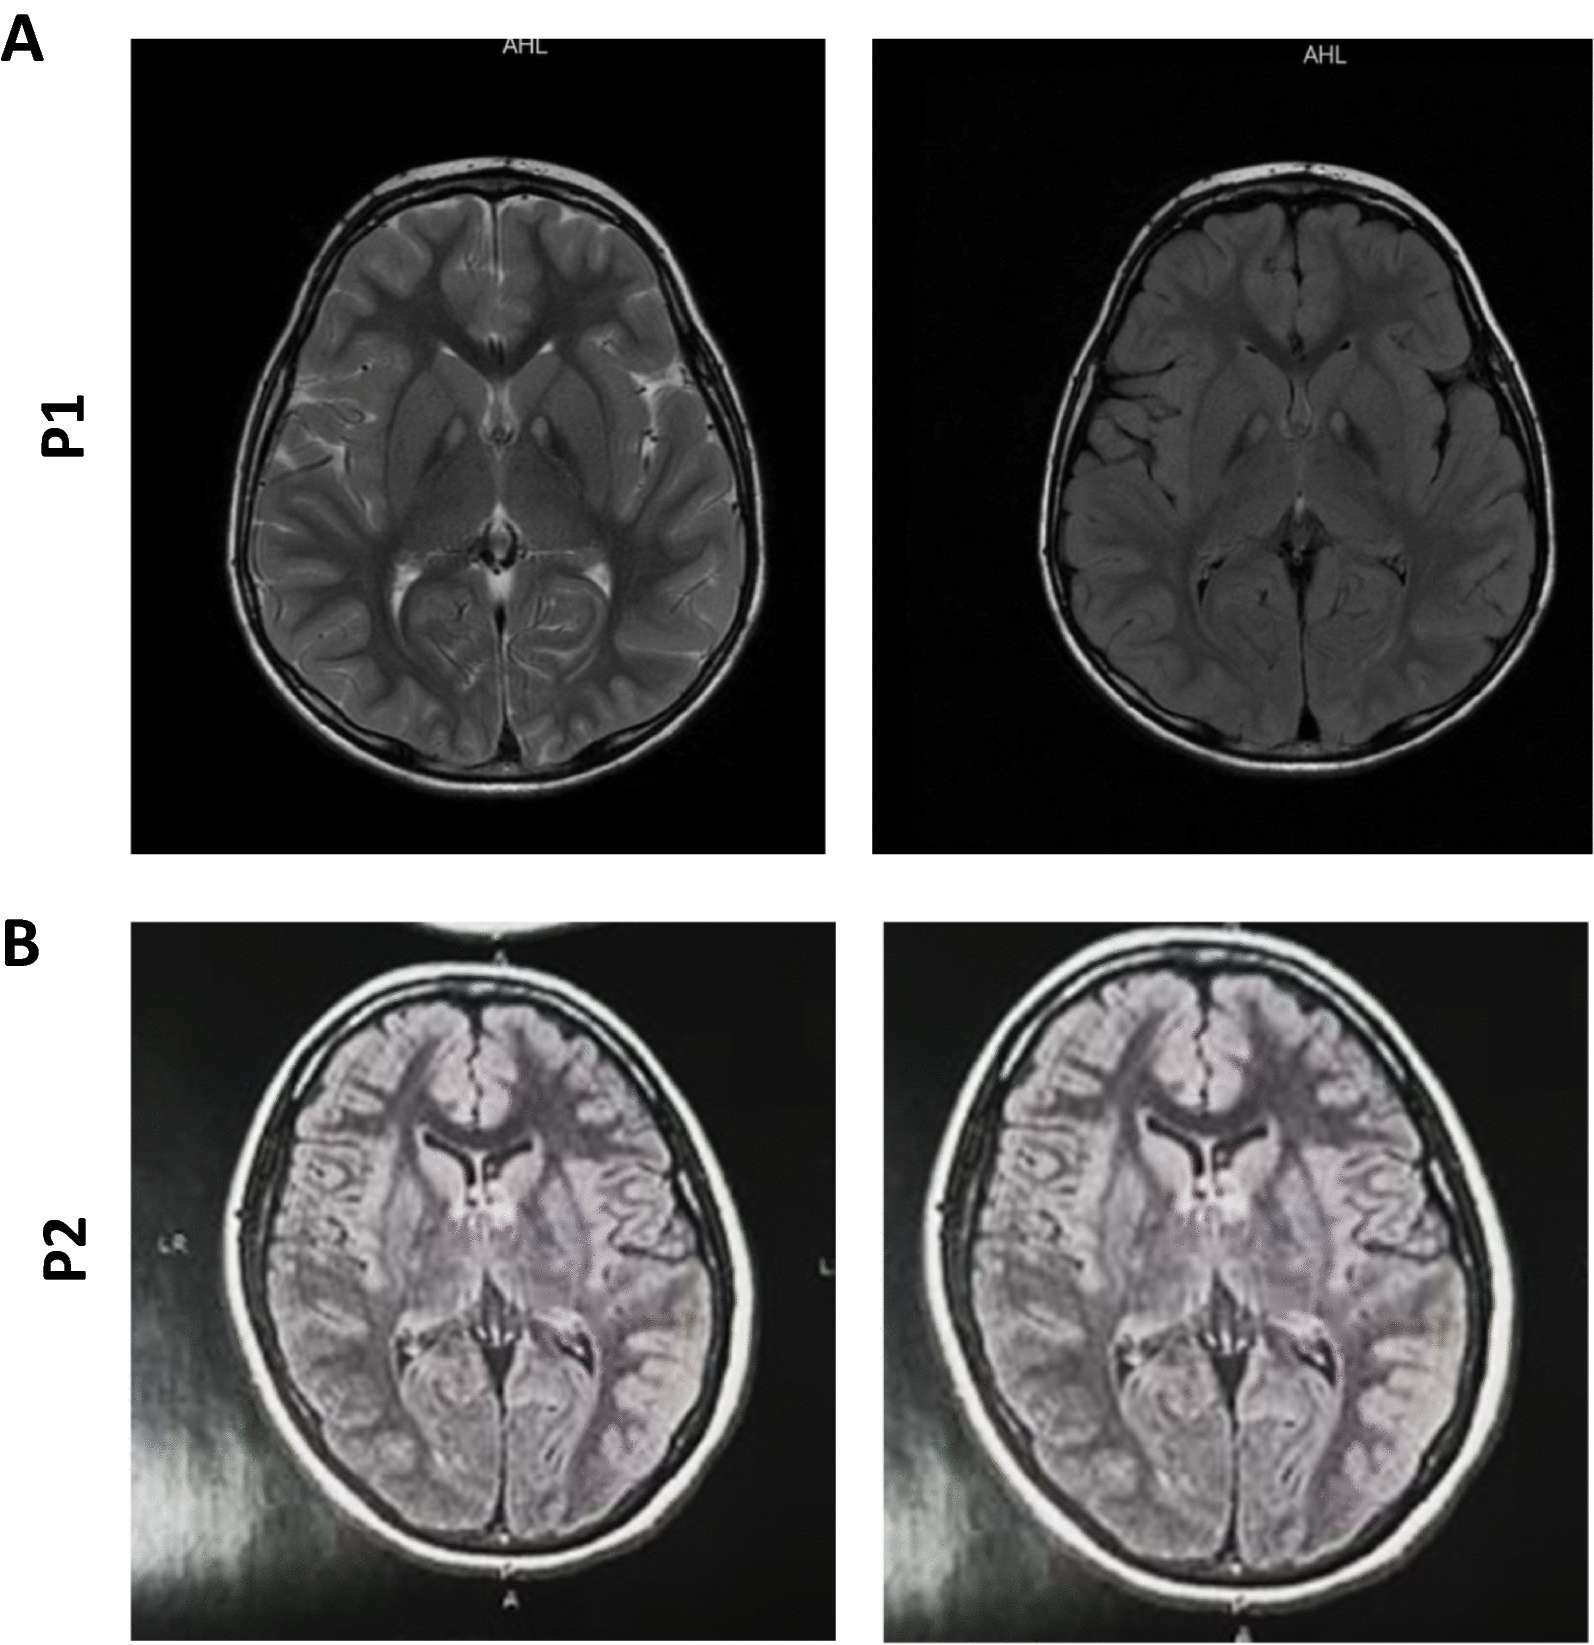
(A) P1: MRI (T2 flair and T2 FSE) showing signal hyperintensity due to gliosis in the antero-medial region of both globus pallidus, surrounded by hypointense areas secondary to iron deposits (“eye-of-the-tiger” sign). (B) P2: Presence of hypersignal in FLAIR sequence, affecting the globus pallidus bilaterally, delimited by a hyposignal halo, more evident in the sequence for magnetic susceptibility and diffusion, characterizing a "eye-of-the-tiger" appearance
